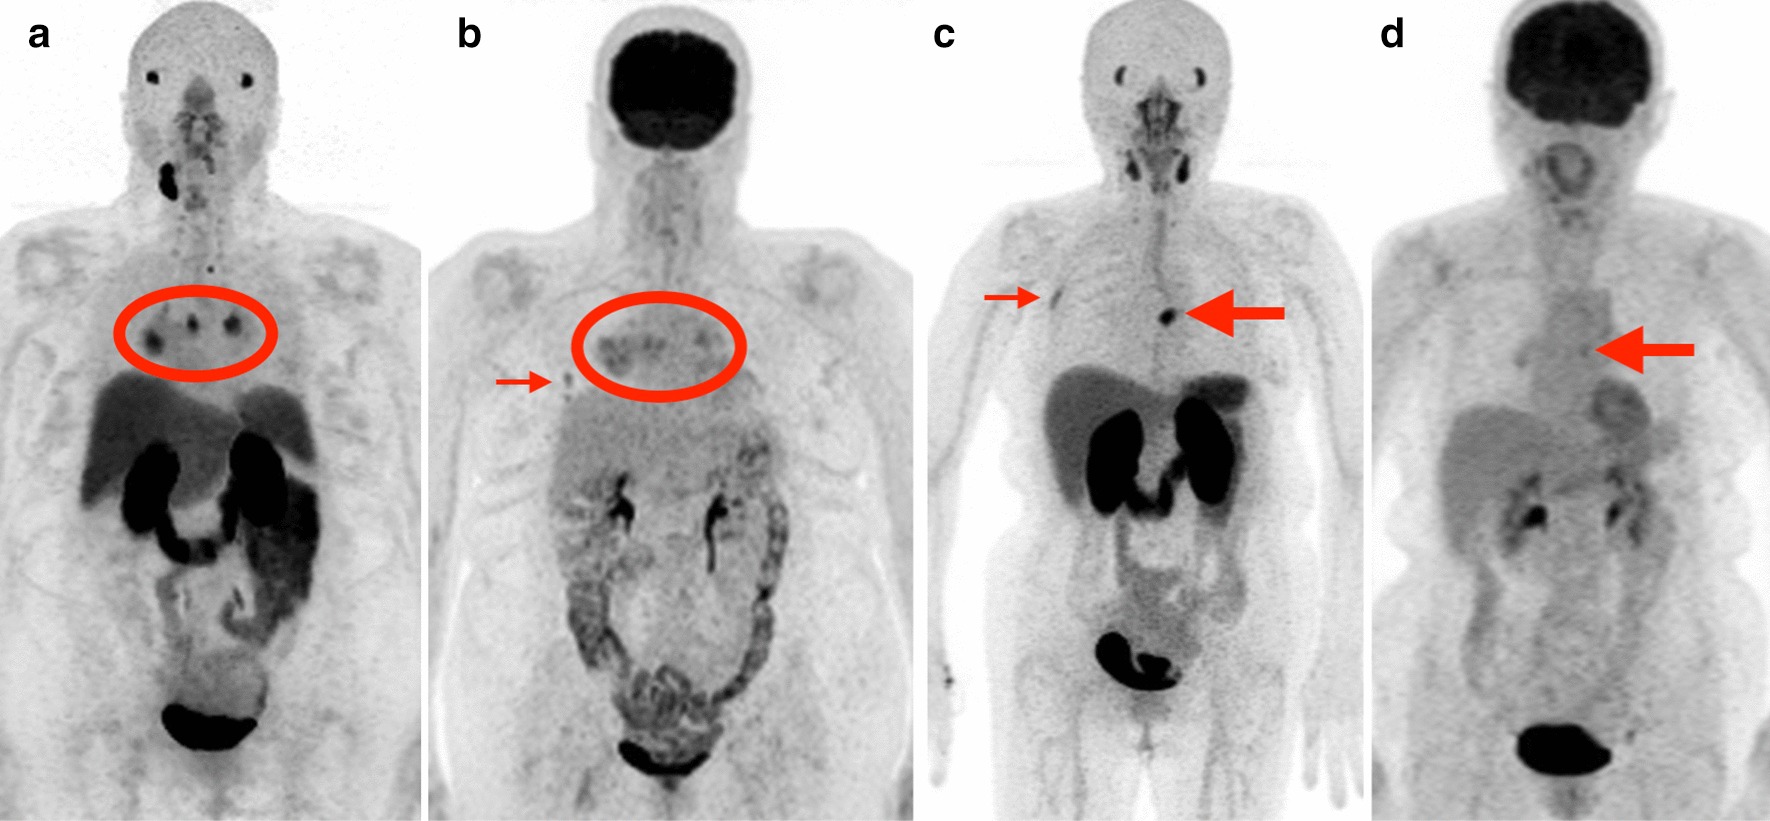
Patients with metastatic thyroid cancer with greater PSMA uptake than FDG uptake: examples. a, b [^68^Ga]Ga-PSMA-11 PET MIP (a) and 2-[^18^F]FDG PET MIP (b) in a patient (Patient 6) with poorly differentiated papillary thyroid carcinoma. Bilateral hilar and mediastinal metastases (circled) are more avid on PSMA (median SUVmax 8.5) than FDG (median SUVmax 4.1). However, a small right middle lobe metastasis seen on FDG (B, small arrow) is not visible on PSMA. C–D: [^68^Ga]Ga-PSMA-11 PET MIP (c) and 2-[^18^F]FDG PET MIP (d) in a patient (Patient 5) with follicular thyroid carcinoma. A left 8th costovertebral junction metastasis (large arrows) is more avid on PSMA (SUVmax 15.0) than on FDG (SUVmax 3.5). In addition, a focus of uptake in the lateral right 4th rib (c, small arrow) is only visible on PSMA PET and may represent an additional site of metastatic disease
